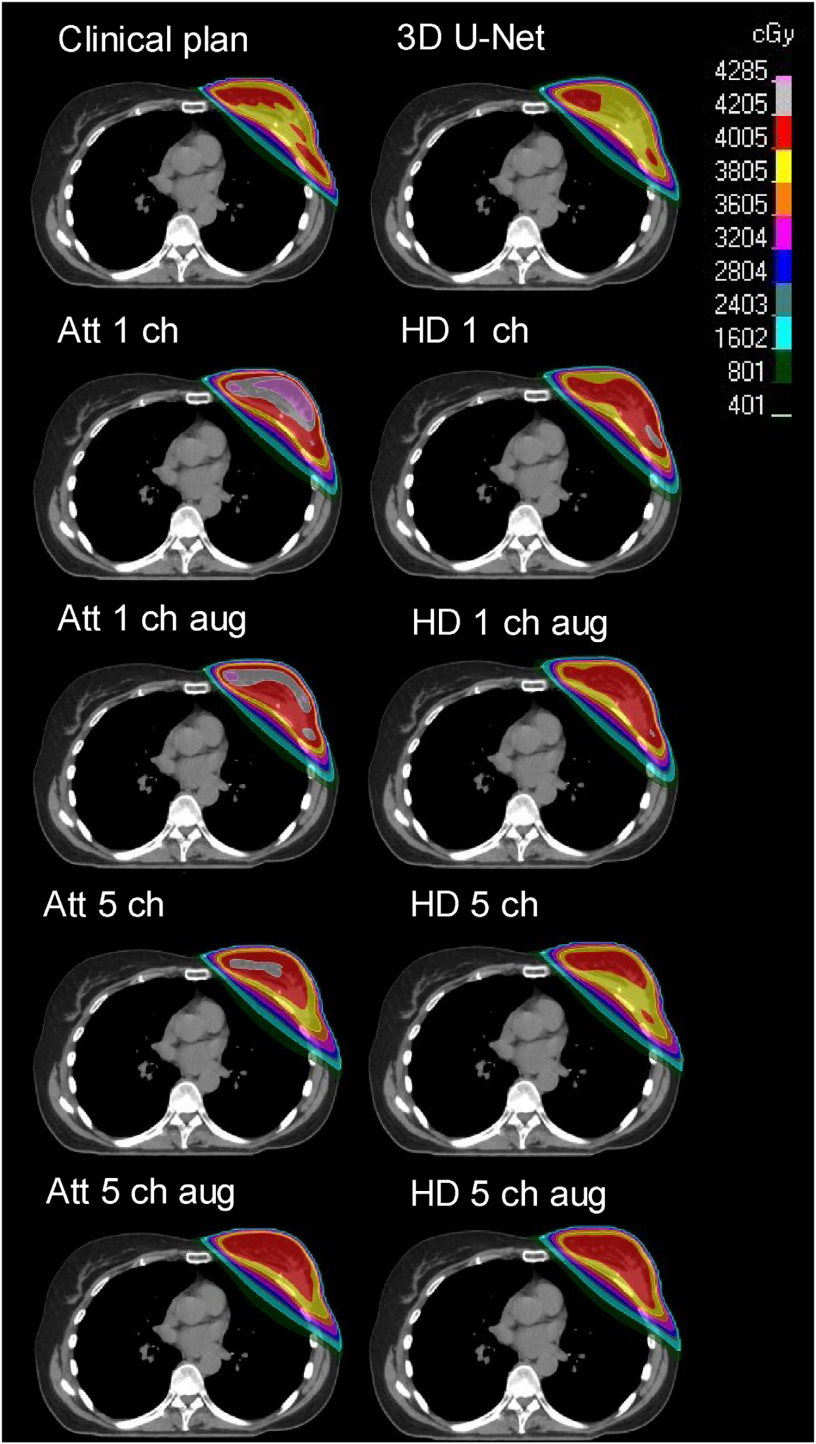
Example of dose predictions from all trained models and the clinical plan in an axial CT slice of one patient.
